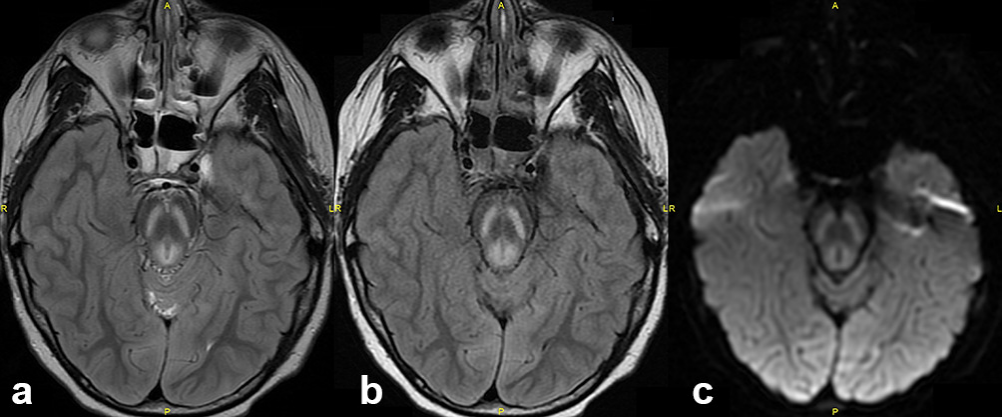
Axial *T*_2_ weighted (a) and FLAIR (b) MRI images of a 24-year-old female who suffered hypoxic brain injury. These demonstrate symmetrical high signal intensity in the mid-brain, thalami and hippocampi. In the mid-brain, the abnormal signal intensity affects the substantia nigra and the periaqueductal grey matter with sparing of the red nuclei and cortical spinal tracts. Diffusion weighted imaging (c) demonstrates increased high signal intensity corresponding to affected regions. FLAIR, fluid attenuation inversion recovery.
